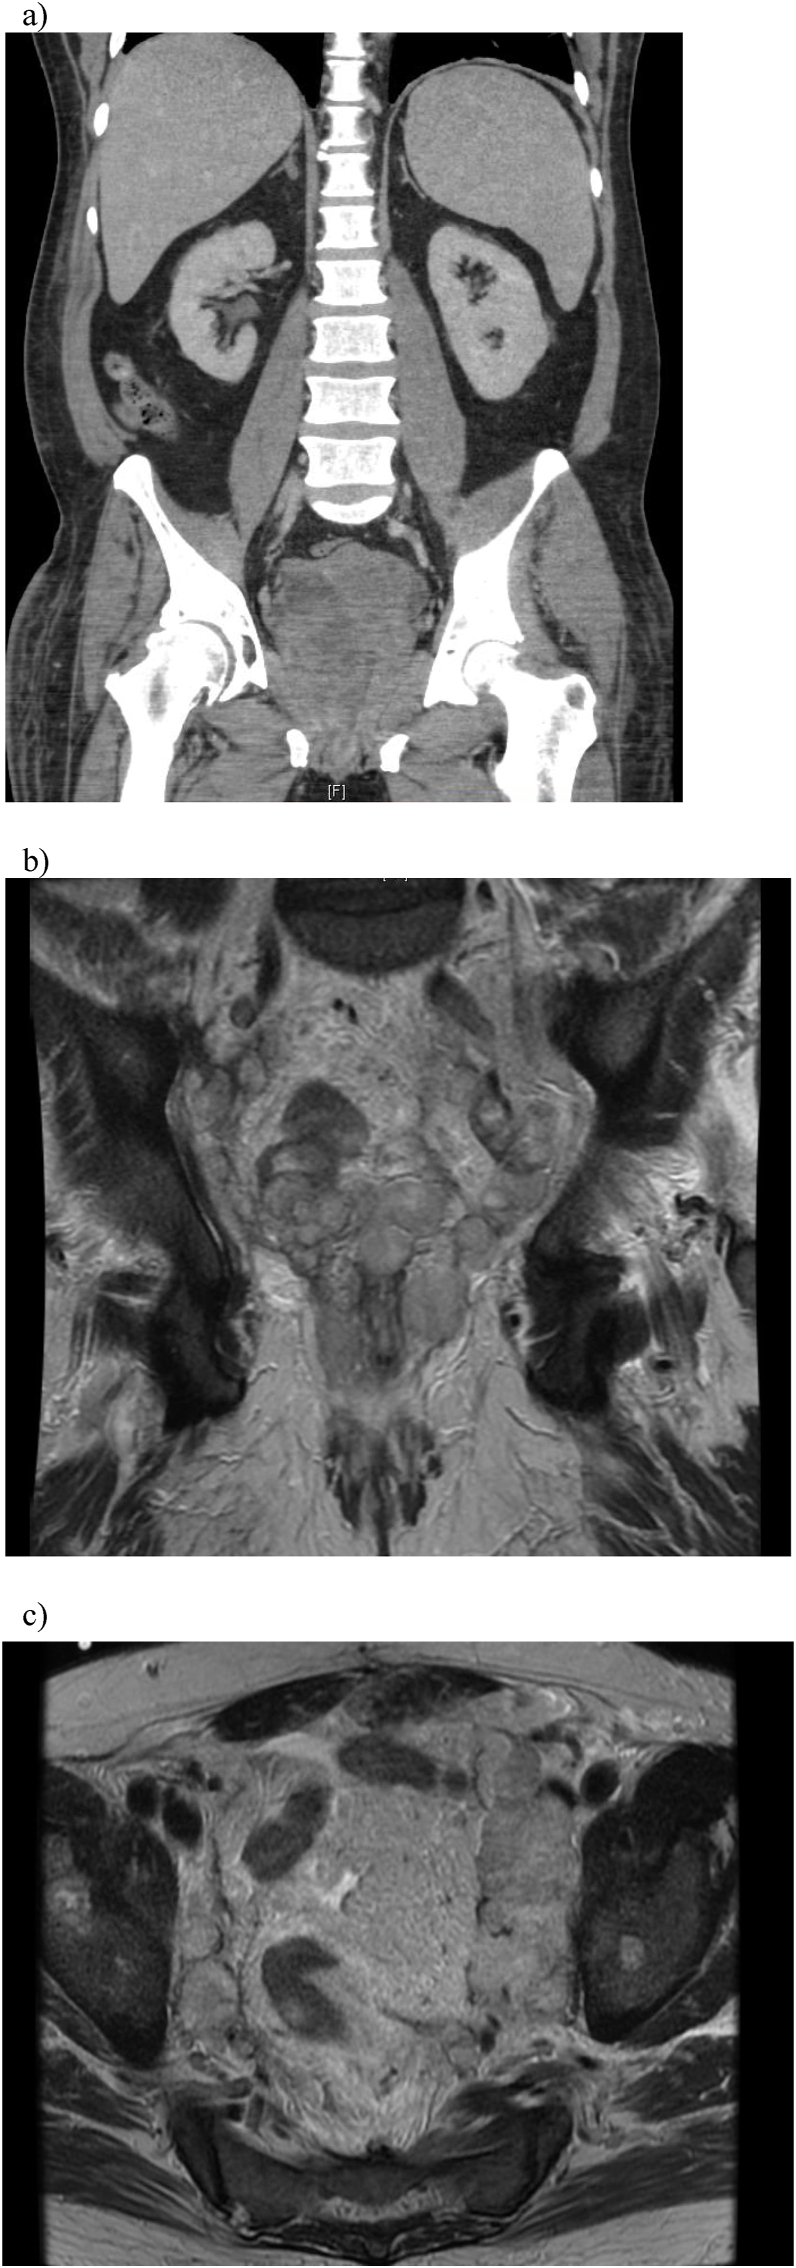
a) Initial CT abdomen/pelvis demonstrating a 776g prostate with highly unusual features. 1b, 1c) Coronal and axial view of MRI pelvis demonstrating recurrent tumor with invasion into the rectal stump and urethra prior to initiation of palliative radiation.
